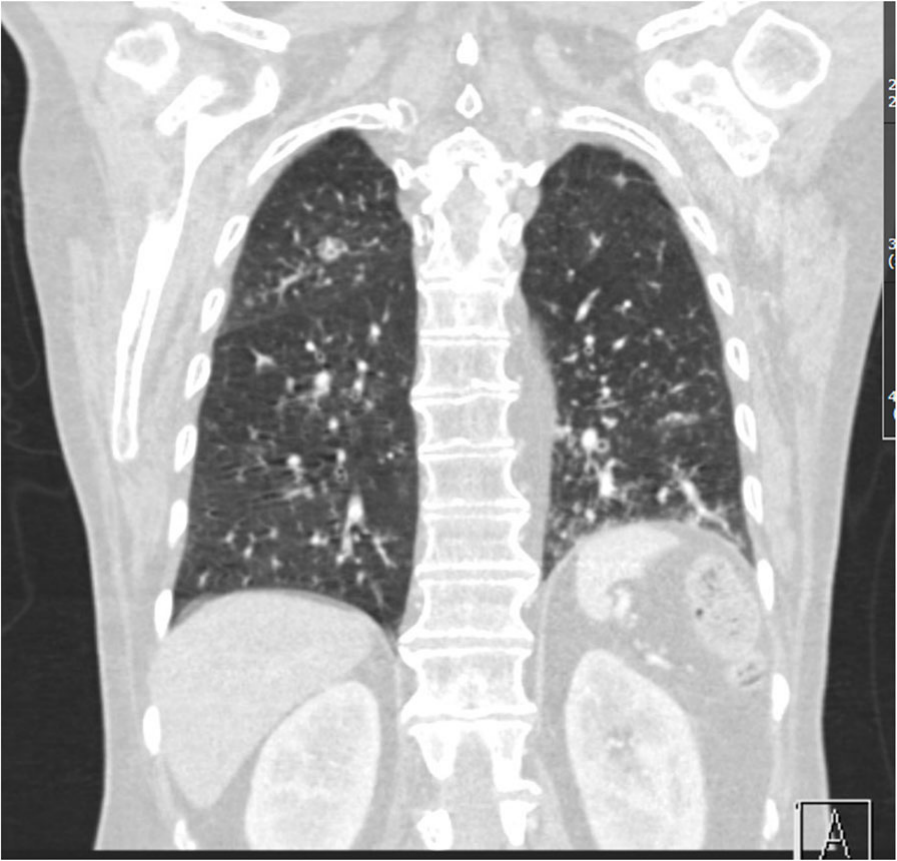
Coronal window CT-scan on day 1 showing pneumonitis with tree-in bud pattern and ground glass opacities
